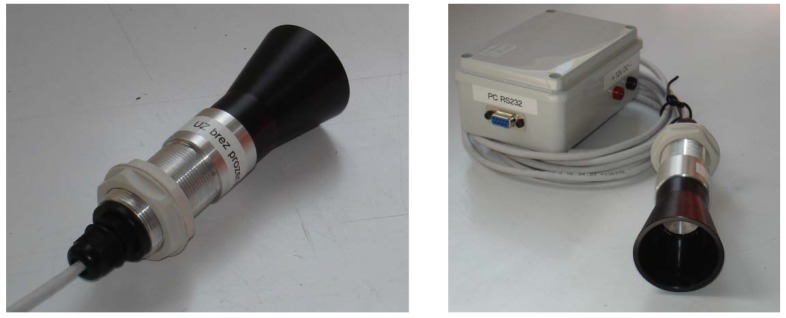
Ultrasonic sensor with ultrasonic transceiver built in the horn. The enclosure includes sensor electronics.
